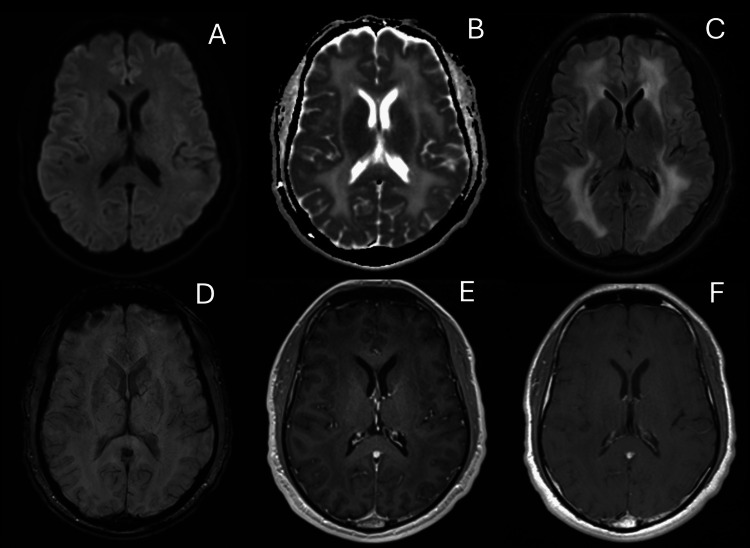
Repeat MRI of the brain Repeat brain MRI, one week after initial presentation. (A, B) DWI, ADC (axial view) without evidence of diffusion-restricting lesions. (C, D) FLAIR, SWI (axial view) demonstrating diffuse white matter signal hyperintensity without concomitant hemorrhage. (E, F) T1-MPRAGE before and after IV gadolinium (axial view) without evidence of gadolinium enhancement. DWI: diffusion-weighted imaging; ADC: apparent diffusion coefficient; FLAIR: fluid-attenuated inversion recovery; SWI: susceptibility-weighted imaging; MPRAGE: magnetization-prepared rapid gradient echo
